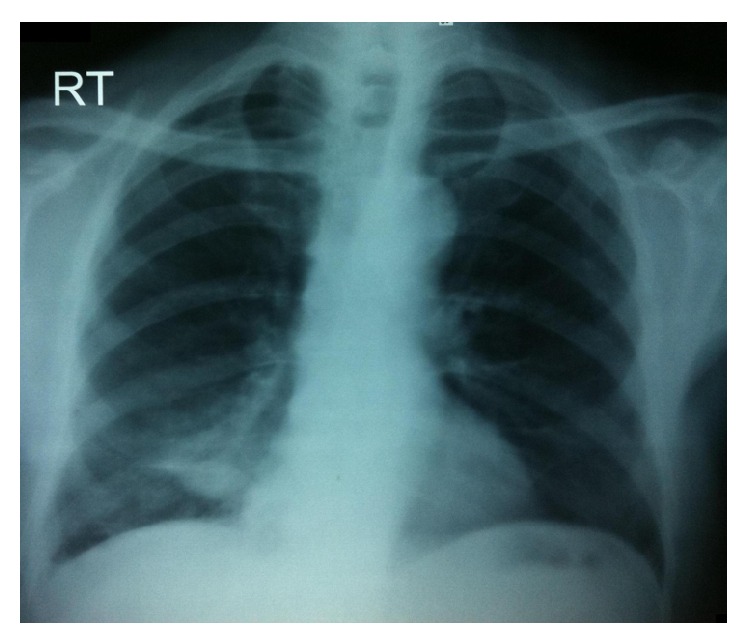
Chest X-ray of the same patient 2 months after pleurodesis and pigtail catheter removal showing no reaccumulation of fluid and successful pleurodesis.
